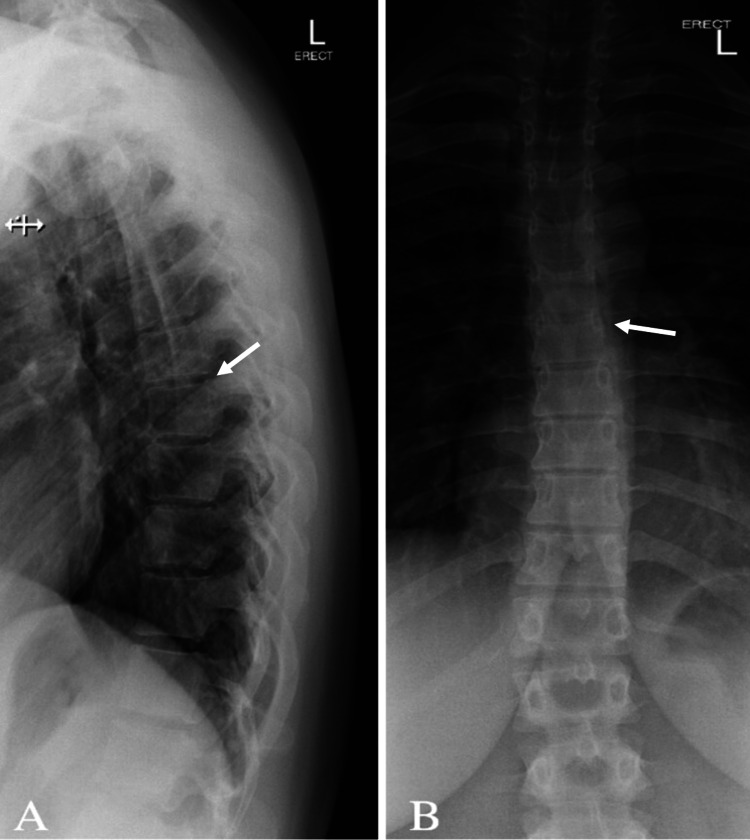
Radiograph of the thoracic spine (A) lateral view and (B) anterior-posterior view demonstrating 12 thoracic vertebrae. Maintained vertebral body heights and disc spaces. No spondylolisthesis. Patent neural foramina.
